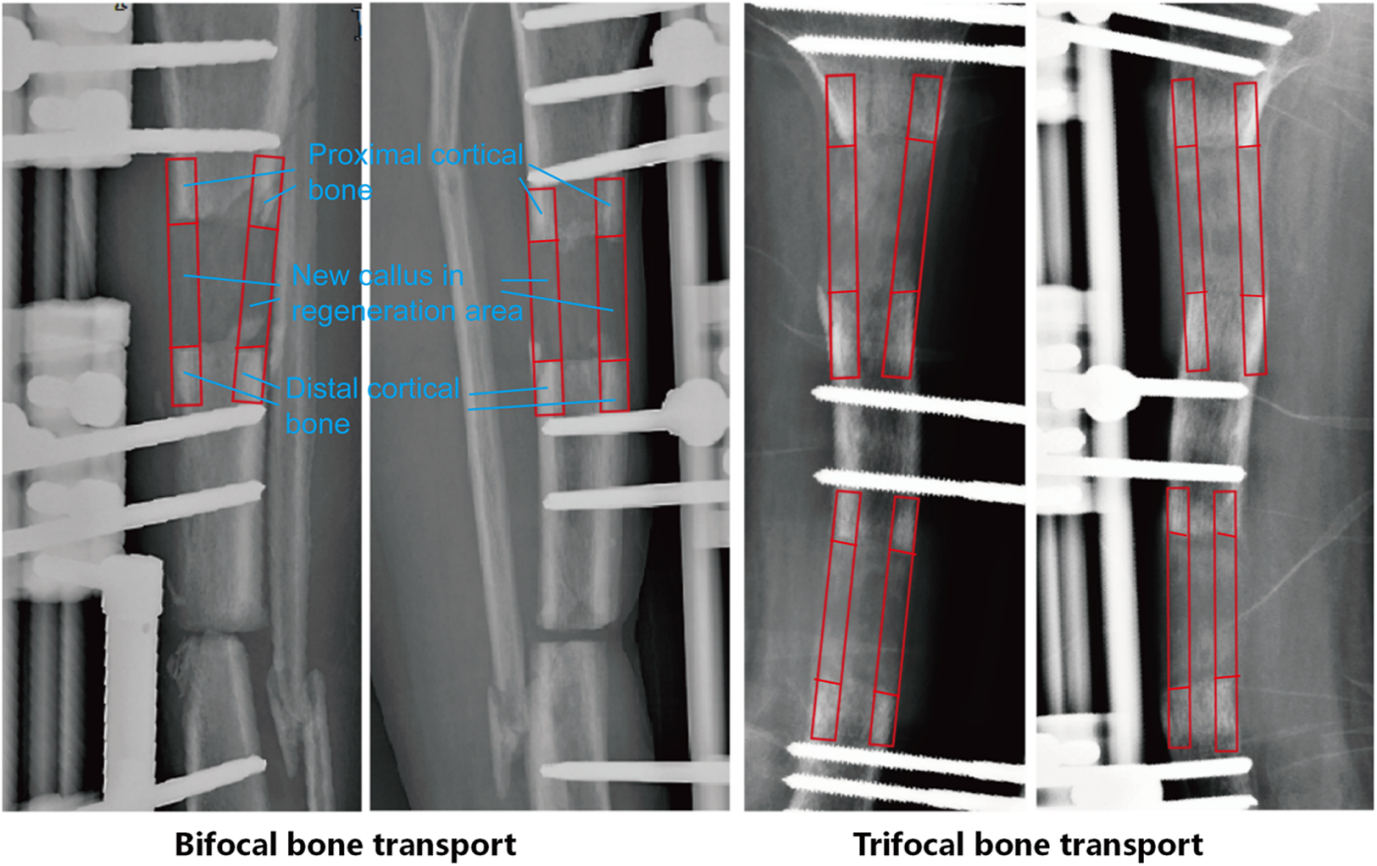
Method for measuring pixel value ratio (PVR) on digital radiographs. Regions of interest (ROIs) are selected in the regenerate bone and adjacent normal bone for PVR calculation.
